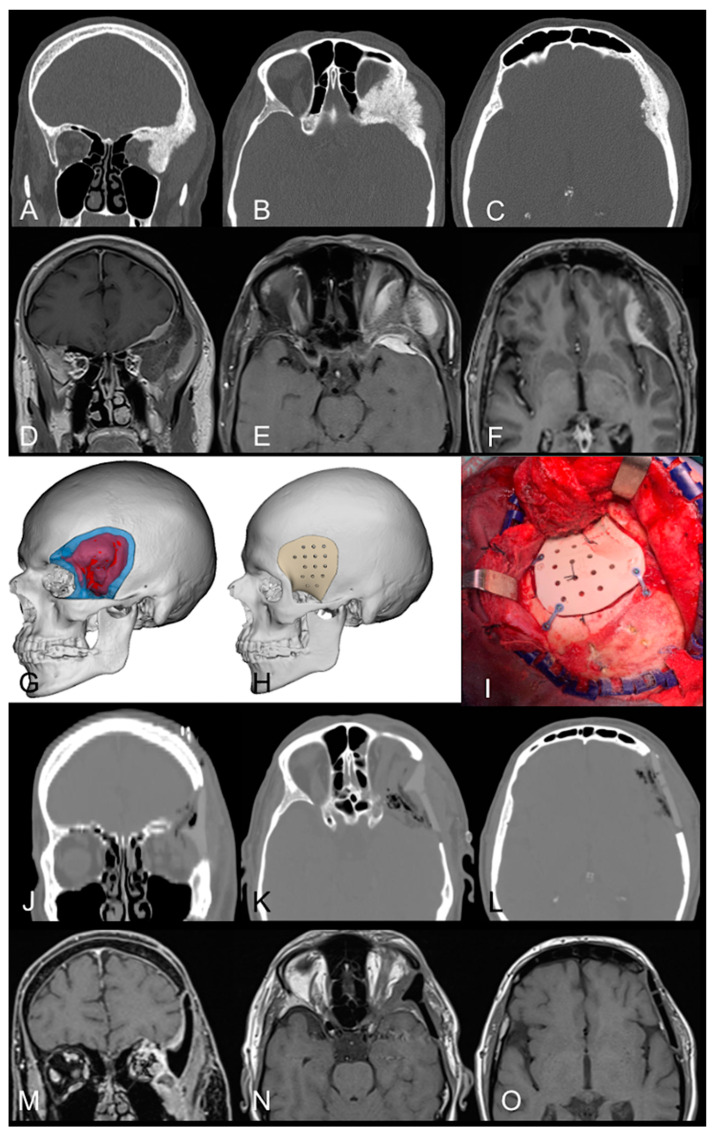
Pre- and postoperative neuroradiology, surgical planning with cutting guide, and intraoperative image. (A–F). Coronal (A), and axial (B,C) CT scans and brain MRI T1-weighted sequence with contrast (coronal—(D); axial—(E,F)) document the left spheno-orbital meningioma with hyperostosis at the level of the superior and lateral walls of the orbit, as well as the frontal bone in the pterional region. The lesion causes significant mass effect on the lateral and superior rectus muscle with exophthalmos and orbital dystopia. (G,H) Three-dimensional digital reconstruction of the CT of the patient, highlighting the extension of the tumor (in red) and the custom-made prosthesis (H). (I) Intraoperative image of the positioning of the implant with the reconstruction of the pterion. (J–O) Immediate postoperative CT scan with coronal (J) and axial (K,L) images. Brain MRI with contrast at four months after surgery (coronal—(M); axial—(N,O)) documents resolution of exophthalmos and temporal swelling and shows a residue into the orbit in tight contact with the lateral rectus muscle.
